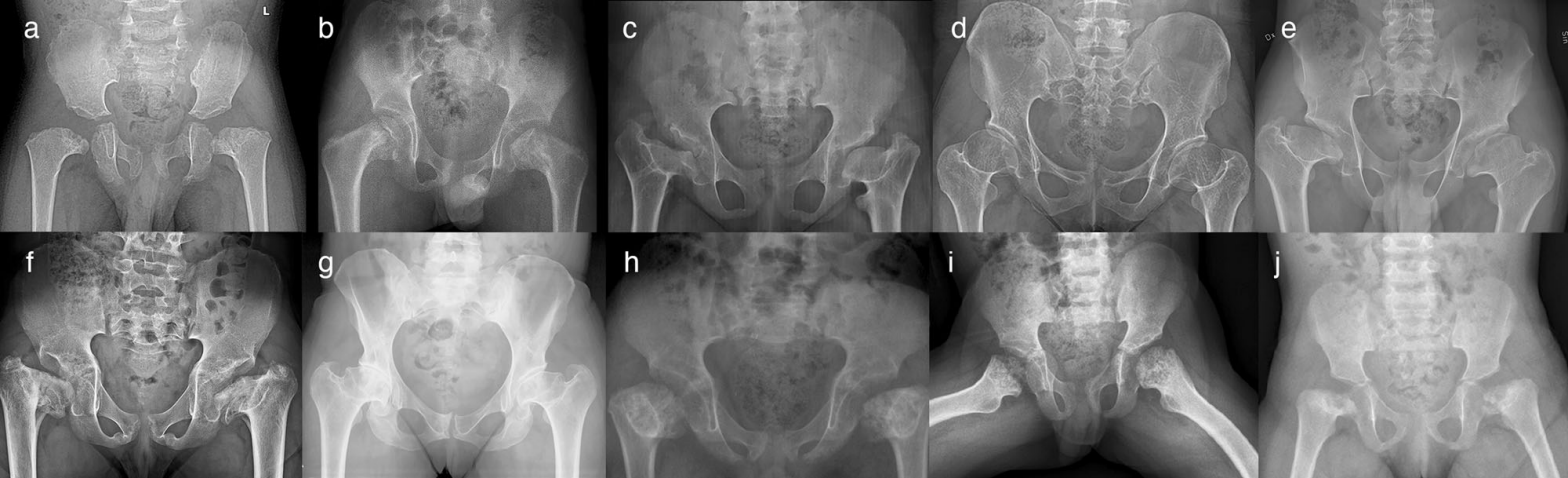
Radiographs of the pelvis. a Patient 1-III:4, radiograph at 3 years; showing unossified capital femoral epiphyses, and metaphyseal irregularities of the proximal femora with short femoral necks and *coxa vara*, and irregular acetabula. b Patient 2-II:2, radiograph at 9 years; showing flat, irregular capital femoral epiphyses, metaphyseal dysplasia of the proximal femora with short necks and *coxa vara*, and normal acetabula. c Patient 3, radiograph at 11 years; showing short femoral neck, severely flat capital femoral epiphyses, severe *coxa vara* and shallow acetabula, together with high-rising greater trochanters. d Patient 4-II:1, radiograph at 25 years; showing short femoral necks with mild *coxa vara*. The capital femoral epiphyses are not deformed, while the joint spaces are narrow. e Patient 4-II:4, radiograph at 15 years; showing severely flat capital femoral epiphyses and short femoral necks with mild *coxa vara* and high-rising greater trochanters. f Patient 5-III:1, radiograph at 10 years; showing short femoral neck, severely flat capital femoral epiphyses, severe *coxa vara*, and shallow acetabula. g Patient 5-II:2 the radiograph at 42 years showing flat capital femoral epiphyses, short femoral necks with mild *coxa vara* and high-rising greater trochanters, and narrow joint spaces. h Patient 6-II:1, radiograph at 7 years; showing short femoral necks, very small and flat capital femoral epiphyses, metaphyseal irregularities of the proximal femora, and severe *coxa vara*. i Patient 7-III:2, radiograph at 6 years 10 months; showing short femoral necks, flat capital femoral epiphysis of the left and delayed capital femoral epiphyseal ossification of the right, and severe metaphyseal irregularities of the proximal femora. j Patient 7-III:3, radiograph at 2 years and 3 months; showing short femoral necks, delayed ossification of both capital femoral epiphyses and severe metaphyseal irregularities of the proximal femora.
